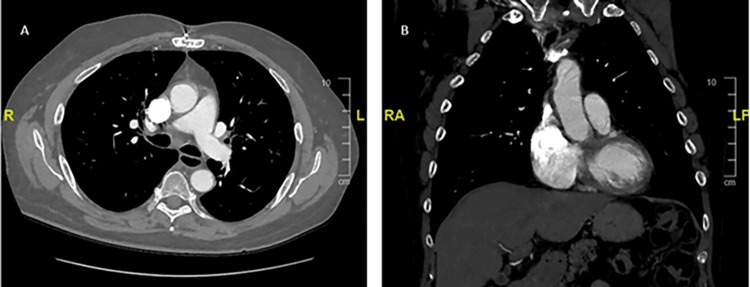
Postoperative computed tomography (CT) angiography. (A) Axial and (B) coronal contrast-enhanced CT imaging four months postoperatively.
